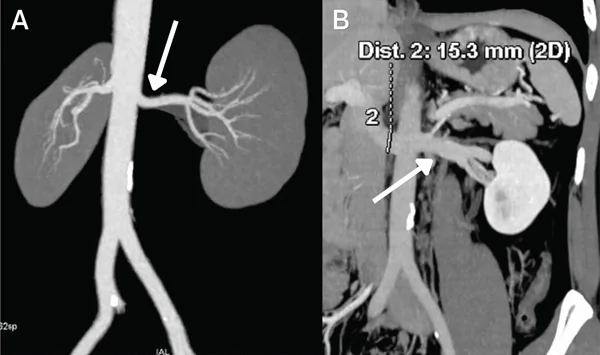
CTA imaging of donor kidney. (A) Left single renal artery (arrow). (B) Left single renal vein (arrow).
